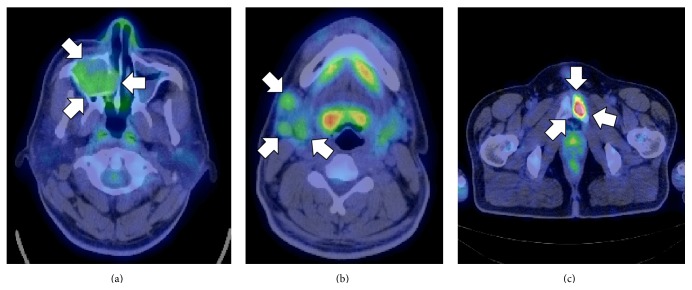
Strong SUVs are found in the right maxillary sinus ((a), arrows), right submandibular region ((b), arrows), and left pubic bone ((c), arrows) on an ^18^F-FDG PET/CT scan.
